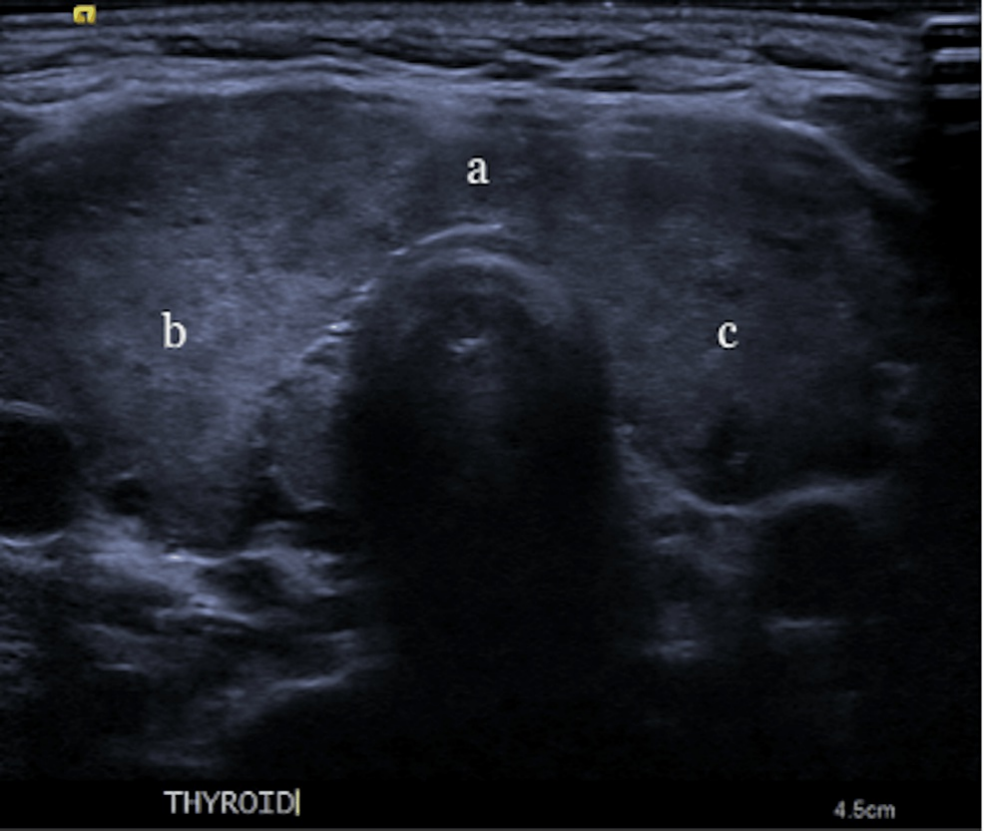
Ultrasound of the thyroid gland Ultrasound of the thyroid gland showing an enlarged and diffusely abnormal-appearing gland with increased echogenicity. The right lobe measures 7.2 x 2.1 x 2.4 cm. The isthmus measures 0.6 cm in thickness. The left lobe measures 6.8 x 1.9 x 2.2 cm. a: Isthmus of the thyroid gland; b: right lobe of the thyroid gland; c: left lobe of the thyroid gland.
